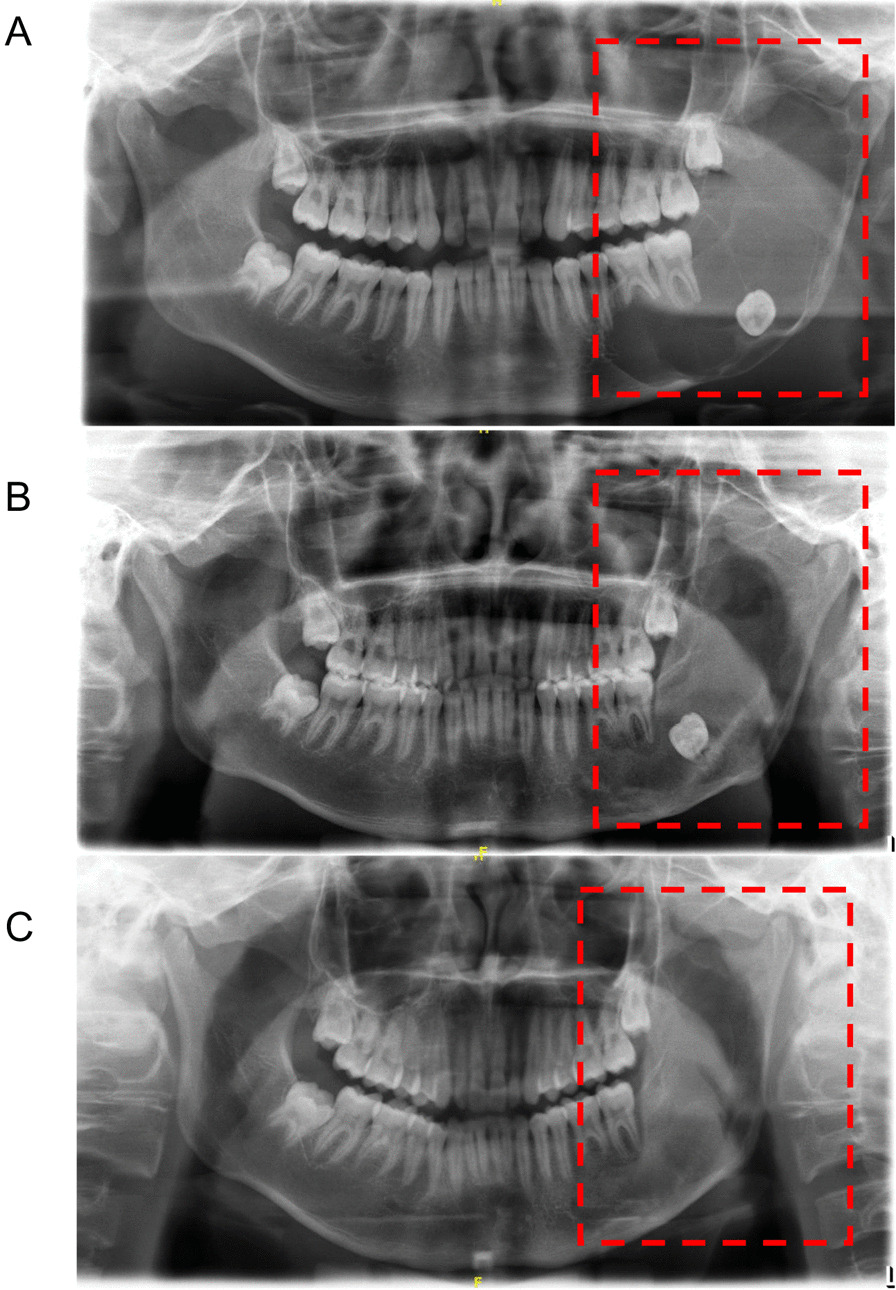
Comparison of pre-operative and post-operative results of FDSC in patients with UA. a Pre-operative panoramic radiography of patients; b The cystic cavity was reduced half a year after fenestration decompression; c The cystic cavity disappeared 2 years after secondary curettage
